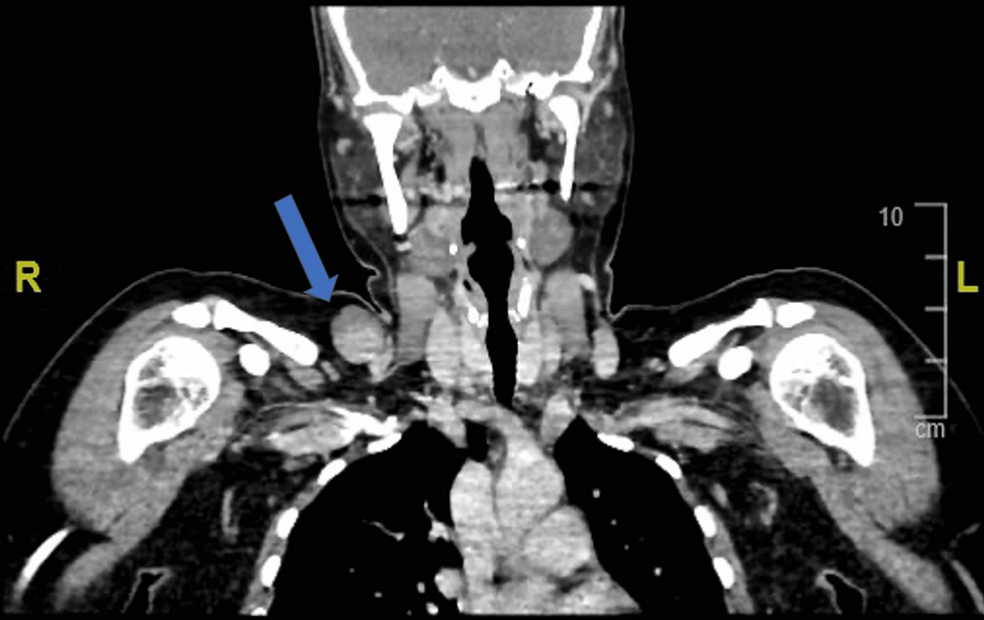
Computed tomography scan of the neck with intravenous contrast (coronal view) revealed a right supraclavicular mass measuring 2.3 x 3.5 cm (arrow). It showed close relation to the right external jugular vein with short neck communication, and it likely represented an external jugular vein saccular aneurysm.
